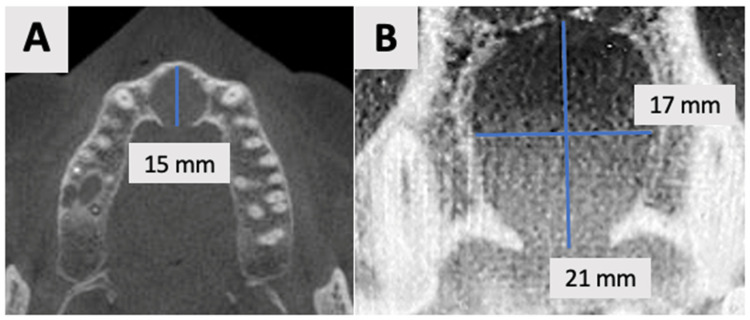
Radiographic images of the cyst at initial visit before marsupialization. (A) Axial, and (B) coronal views of the cyst center. Measurements indicate cyst dimensions: mesiodistal 17 mm × buccolingual 15 mm × height 21 mm.
